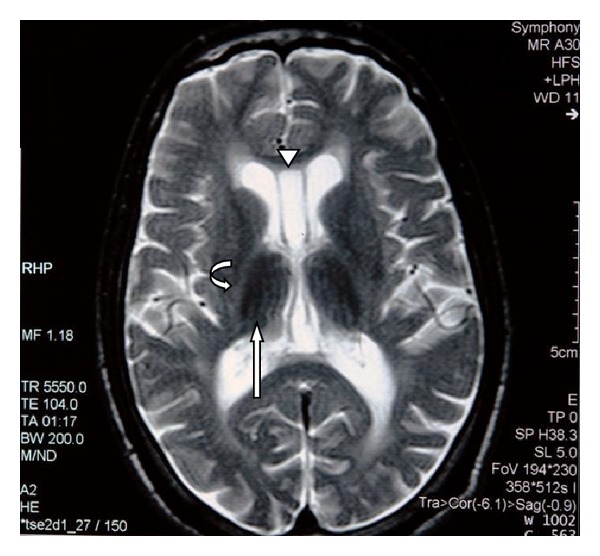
Axial T2 weighted MR image of the brain at the level of the basal ganglia. There is symmetric hypointensity of the thalami (straight arrow) as well as hyperintensity of the posterior limbs of internal capsule (curved arrow). Cerebral atrophic changes also noted with rounded horns of lateral ventricles. Normal variant cavum septum pellucidum and vergae noted (arrow head).
